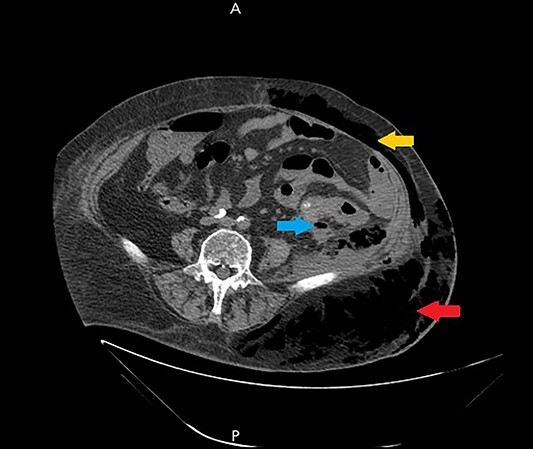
A non-contrast abdomen and pelvis CT scan, which shows a large amount of subcutaneous emphysema within the left flank soft tissues (lower arrow) that wrapped anteriorly along the left lower lateral abdominal wall into the anterior subcutaneous soft tissues of the left lower quadrant (upper arrow). There was also a small tract of air extending from the left retro peritoneum posterior to the left kidney and extending along the left iliopsoas muscle inferiorly (middle arrow).
